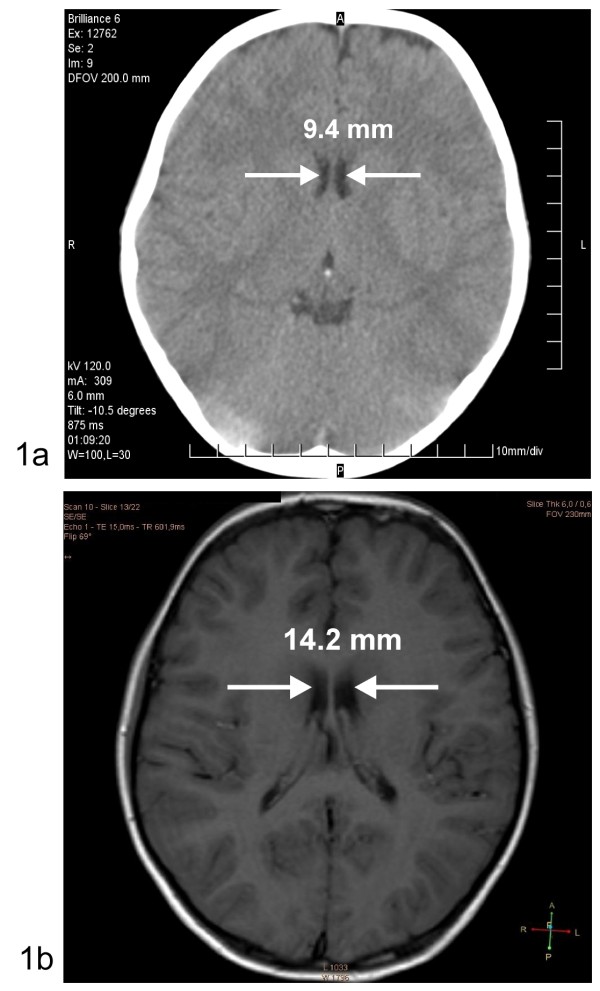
Intercaudate diameter: (a) according to Glaser et al. [15], during DKA. Computed tomography without contrast media; (b) after full recovery from DKA. Magnetic resonance imaging (T1 weighted) without contrast media.
